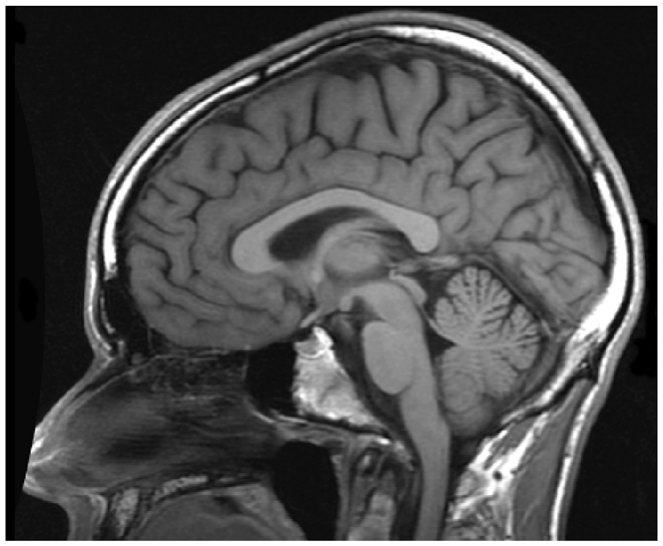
Cerebellar atrophy in Case 3. T1-weighted MRI reveals cerebellar atrophy. Note this is a T1-weighted image (not a T2-weighted image as in Figure 2A); the bright layer in the scalp is diploe, not subcutaneous fat.
